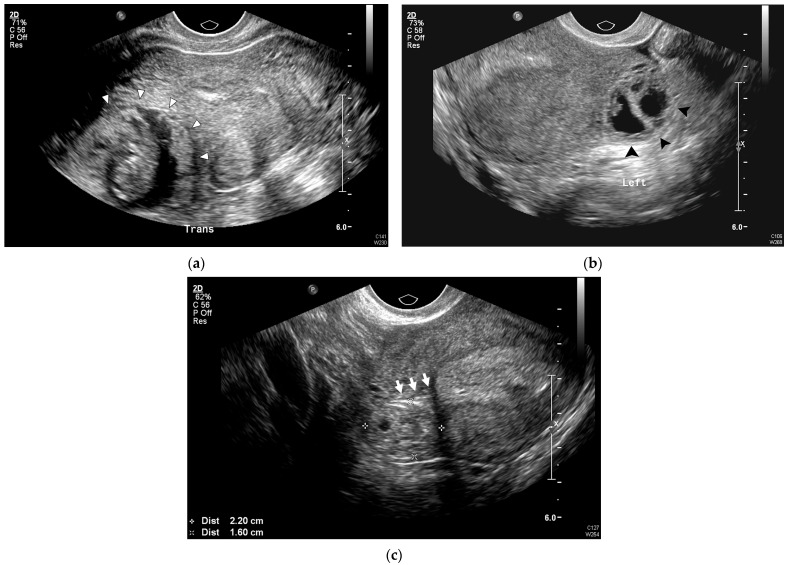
Representative US images of IP. (a) Eccentrically located round ring-like mass (white arrowhead) in the right uterine cornu in the transverse plane. (b) Asymmetric, thin myometrial (<5 mm) layer surrounding the GS (black arrowhead), without surrounding endometrium. (c) Empty uterine cavity and interstitial portion of the tube extending from the uterine cavity to the GS (interstitial line sign, white arrow). GS, gestational sac; IP, interstitial pregnancy; US, ultrasound.
